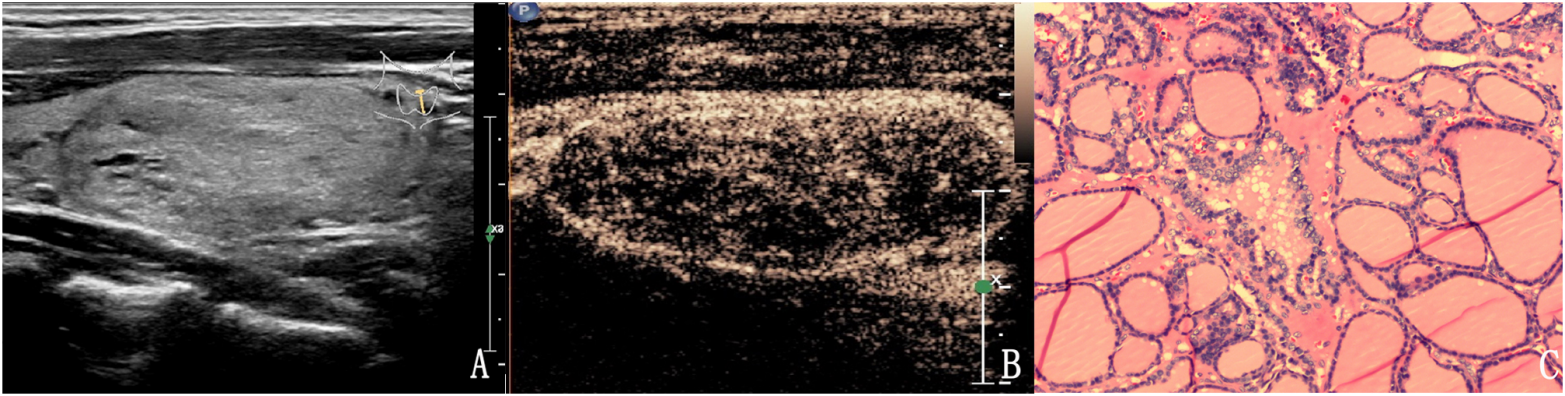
The case of a 46-year-old woman with a 37.3 × 18.1 × 24.7 mm solid hyper-echoic nodule in the left lobe of the thyroid. (A) Conventional two-dimensional image showed that the nodule had one malignant indicator (solid) and was classified with a TI-RADS score of 3. (B) Ultrasound contrast image showing ring enhancement. The modified version of TI-RADS combined with CEUS returned a score of 2 and the patient was diagnosed with a benign nodule. (C) Pathological image of the lesion, a nodular goiter.
